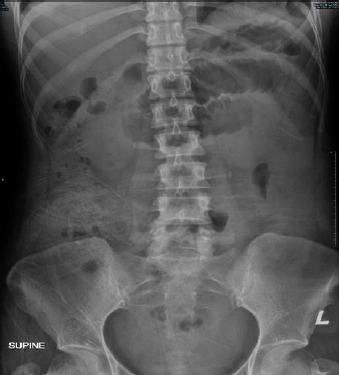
Abdominal radiography showed prominent small bowel loops.
